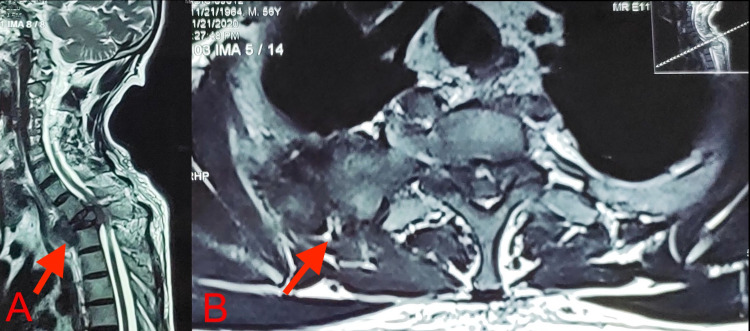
T2-weighted sagittal (A) and axial (B) MRI revealed the collapse of the T2 vertebral body and adjacent hypointense soft tissue component extending to the epidural space compressing the cord and paravertebral region creeping along the right second posterior rib. MRI: Magnetic Resonance Imaging
